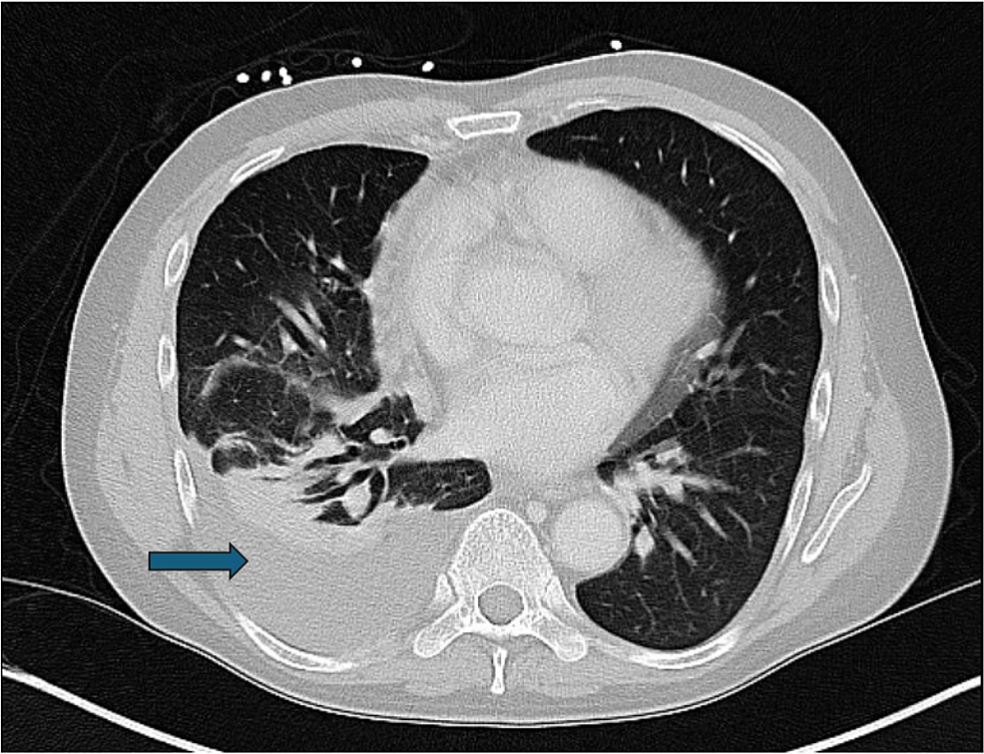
A repeat CT scan of the chest reveals the recurrence of a small-to-moderately-sized right-sided pleural effusion.
